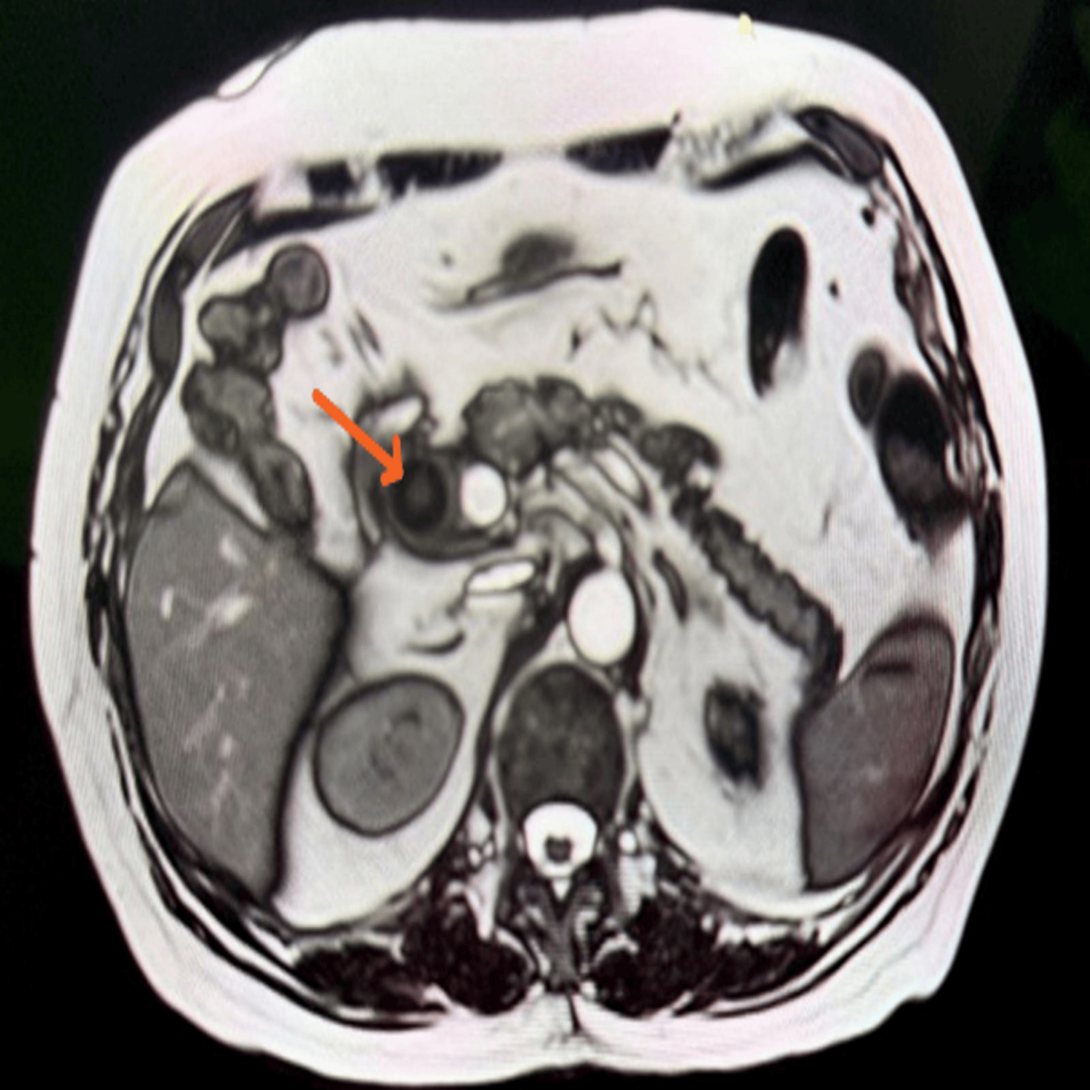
Axial T2-weighted MRI image showing an intraductal filling defect in the region of the mid common bile duct (CBD), suggestive of an intraductal calculus
